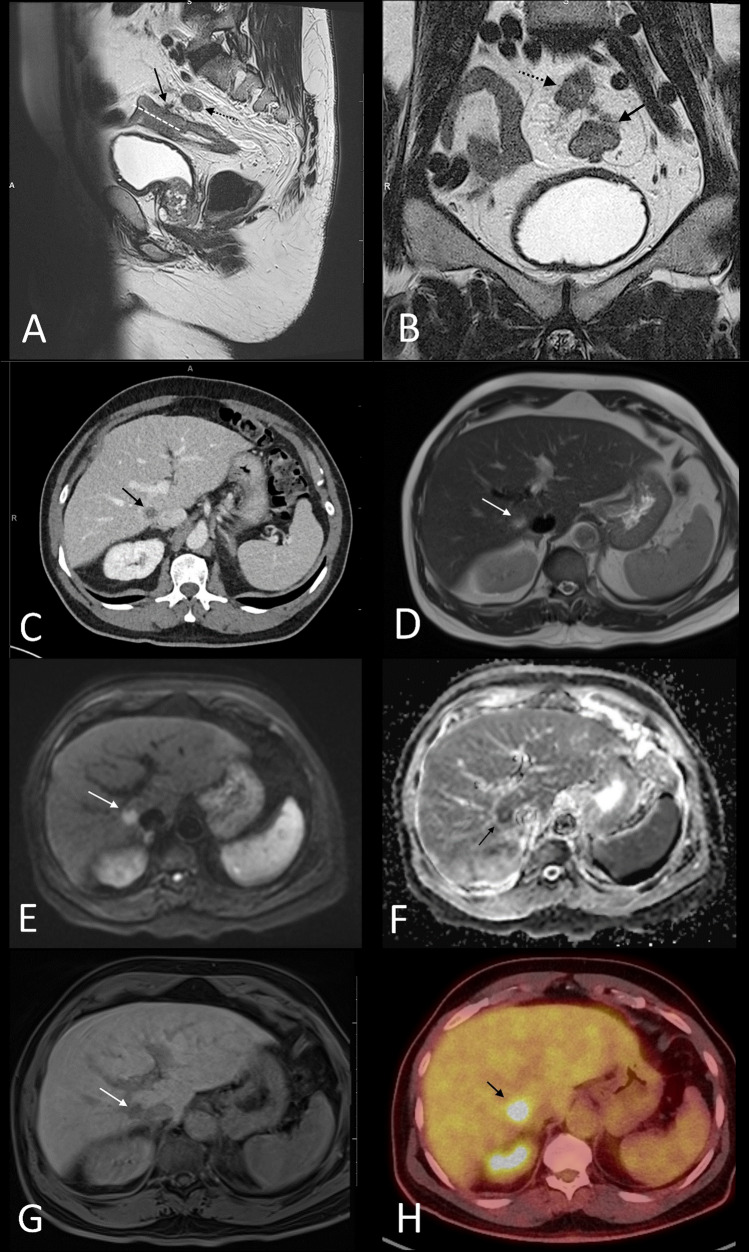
A and B sagittal and high-resolution coronal oblique images of a high-risk T3c N1c EMVI-positive CRM-positive PSW-negative upper rectal tumor; dashed white line delineates the maximal length of the tumor, solid black arrow the EMVI, and dashed black arrow the vascular deposit (N1c); C contrast-enhanced CT showing a hypodense lesion in the right lobe of the liver but without definite peripheral enhancement to confirm the presence of liver metastasis; D T2-weighted MRI of the liver showing the lesion to be high signal; (E) and (F) B800 series of the diffusion weighted sequence, E showing the liver lesion to be high signal with corresponding low signal on the ADC map and F confirming the lesion restricts diffusion; G 25-min (delayed) hepatocyte specific contrast-enhanced MRI showing wash-out confirming the lesion to be a metastasis, no further metastases are demonstrated; H PET-CT showing the lesion is FDG avid again, confirming the lesion to be a metastasis; in this case abbreviated liver MRI confirmed the lesion identified on CT to be suspicious for a metastasis and that there were no other suspicious liver lesions
